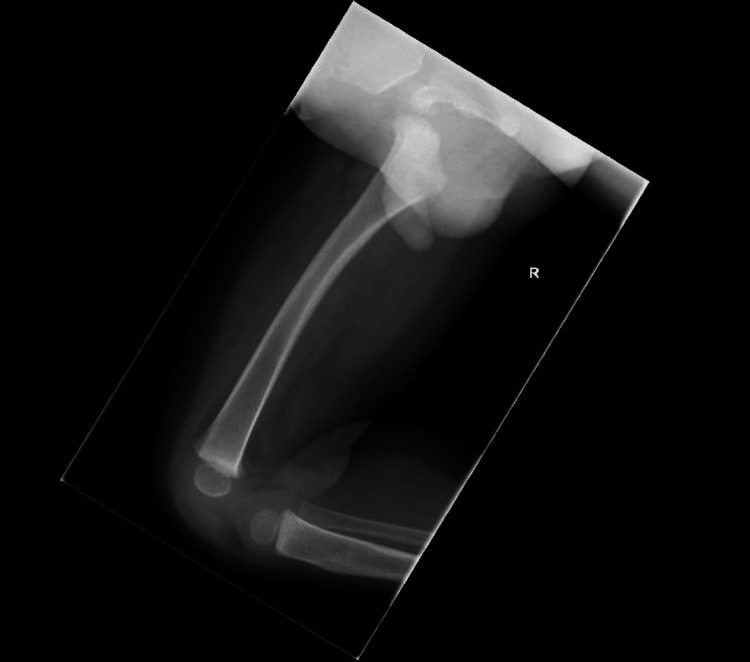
An X-ray of the femur with a lateral view shows no acute femoral fracture or focal bony lesion. Normal alignment is preserved at the knee joint.
